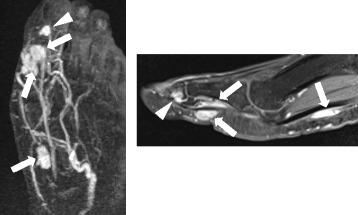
Magnetic resonance imaging. Gadolinium-enhanced T2 weighted magnetic resonance imaging shows three soft tissue tumor presented along right flexor hallucis longus muscle (*arrow*) and one tumor in right first distal phalanx (*arrowhead*)
